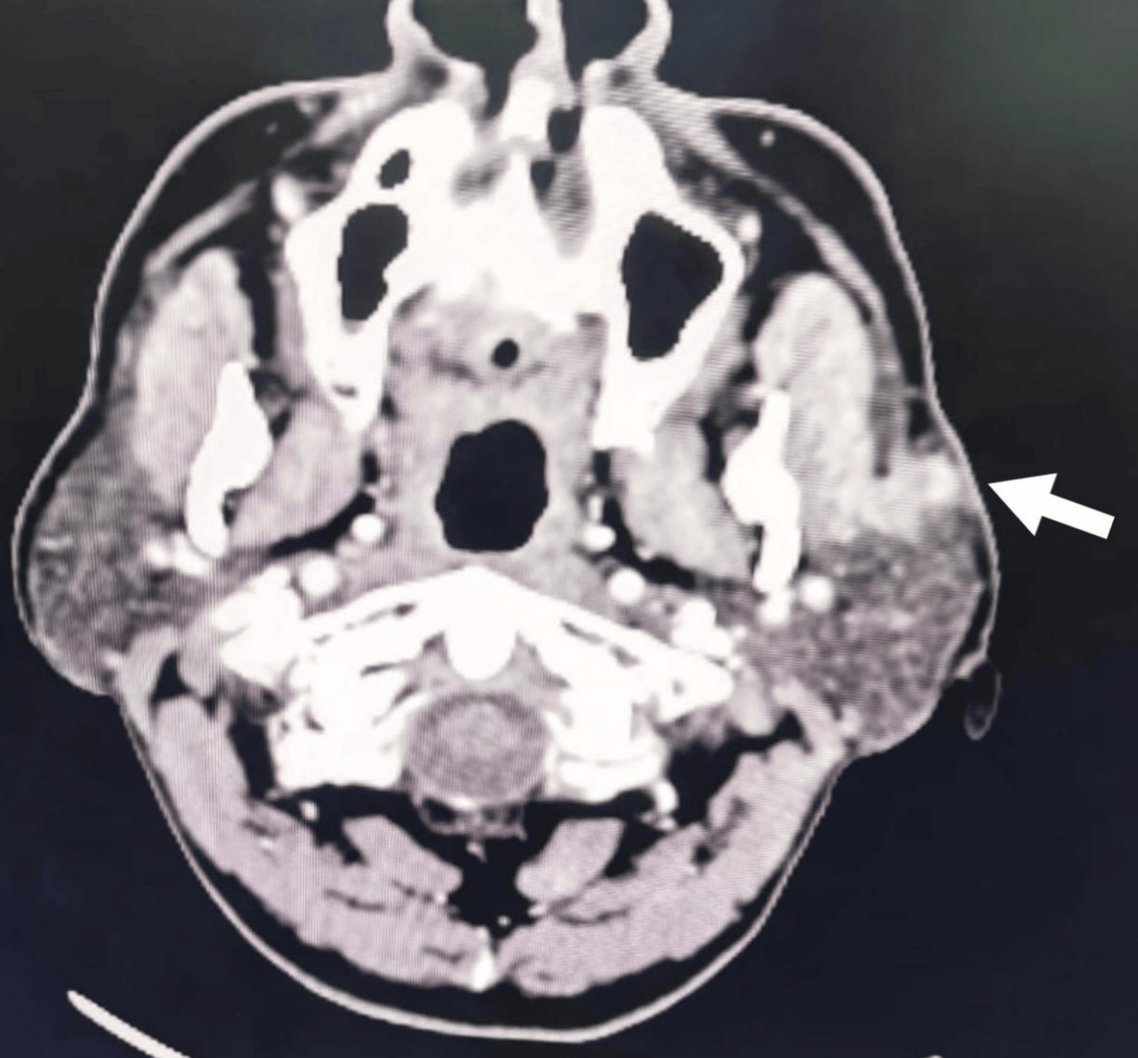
CECT neck showing a heterogenous enhancing soft tissue density lesion of 10*15*12mm size in the superficial lobe of the left parotid gland suggestive of pleomorphic adenoma of the left parotid gland marked by a white arrow. CECT: Contrast-enhanced computed tomography
